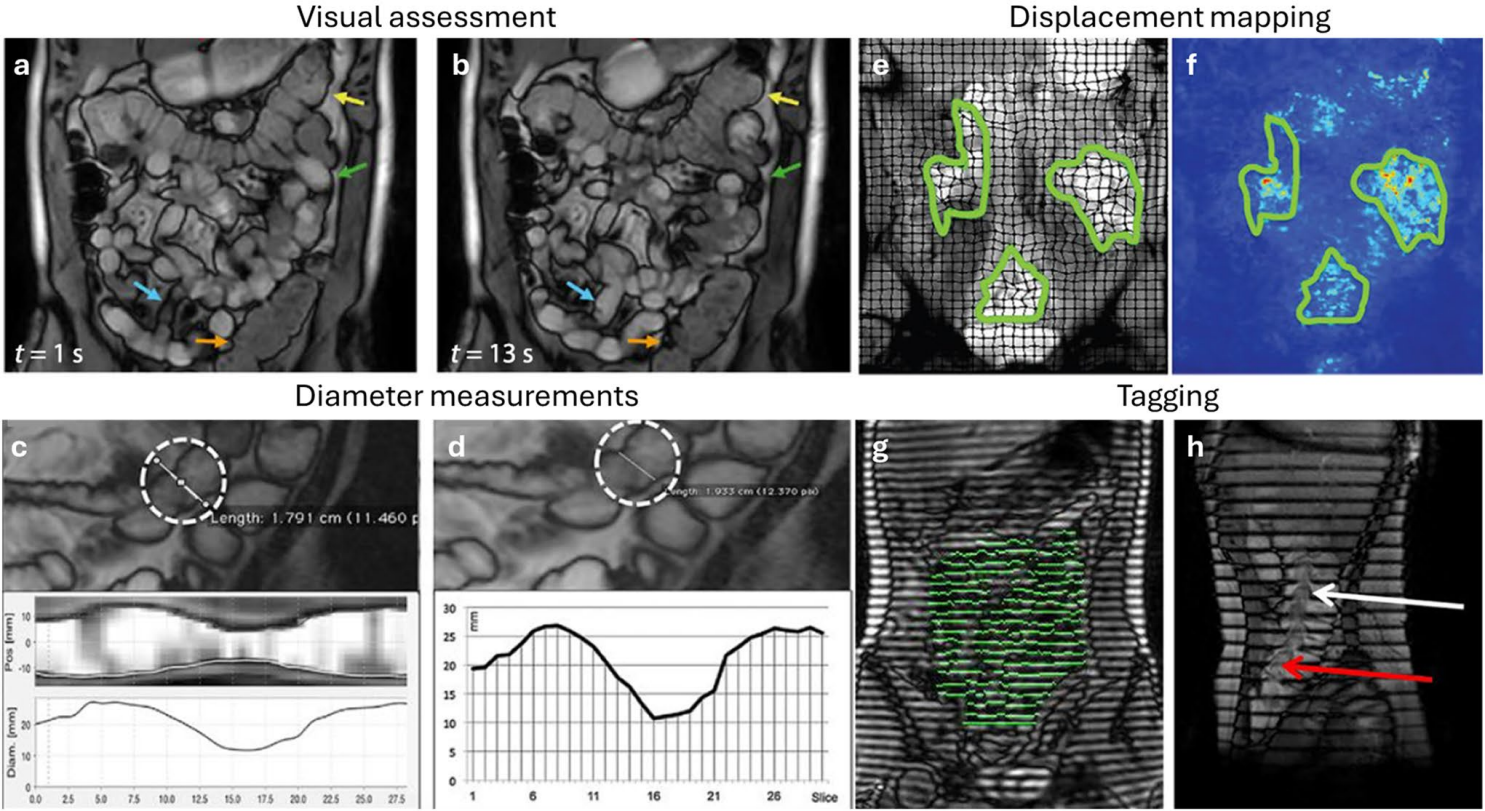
Peristaltic motion in gastrointestinal tract (GI) imaging. (a and b) Dynamic MRI coronal images visualize the GI tract movement over time. Arrows point to different structures: Stomach (red), small bowel (green, blue), and colon (yellow, orange). (c and d) Small bowel diameter measurements can be performed using software assistance (c) or manually with assistance (d). (d–f) Coronal tagging allows for visualization of deformation fields and calculation of displacement. In (g) a coronal GI tagged image is visualized showing deformed taglines in the small bowel n green. In (h) a sagittal image shows movement within the colonic chime, with tag distortion (white arrow) and reduction in tag intensity (red arrow) due to movement. Adapted from [61].
